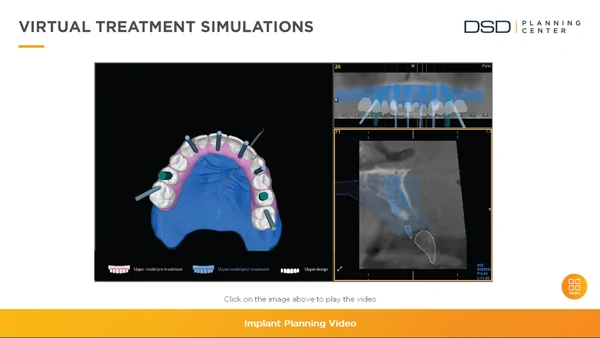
A cone‐beam CT (CBCT) scan was obtained to evaluate bone volume for implants.
